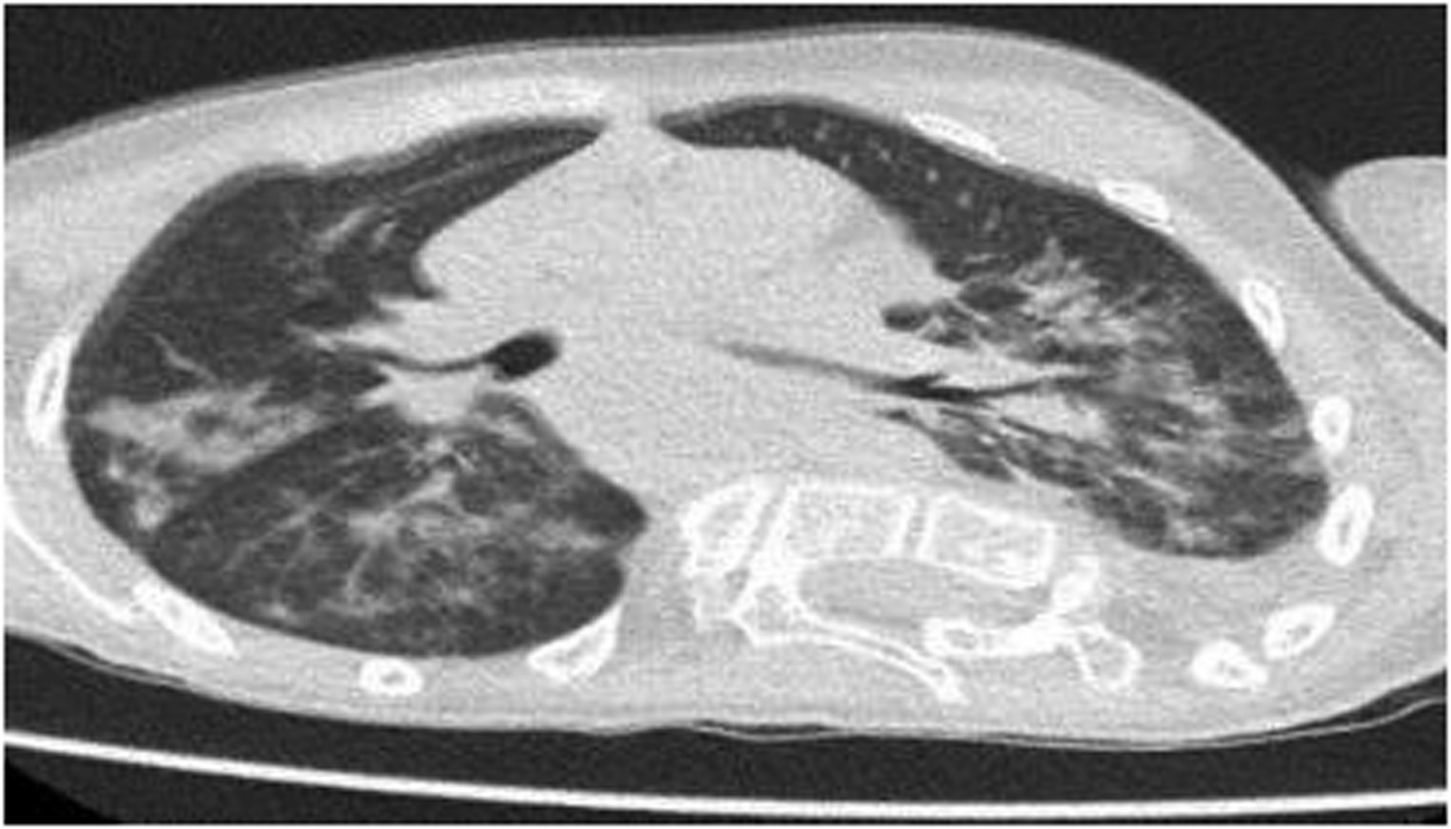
High-resolution computed tomography (HRCT) of a 12-year-old boy with Canavan disease, epilepsy, and COVID-19 pneumonia: Bilateral areas of diffuse alveolar consolidation and infiltration that are more pronounced in the upper lobes and sporadic ground-glass opacities. COVID-19: coronavirus disease 2019.
